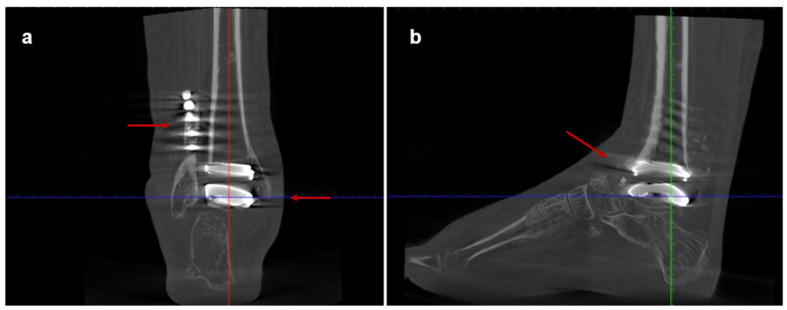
Postoperative weight-bearing computed tomography (WBCT) scans of Case one: (a) coronal and (b) sagittal views at 3-year follow-up. The arrow in panel (a) indicates correct implant positioning and stable fixation, while the arrow in panel (b) indicates absence of radiographic signs of loosening.
